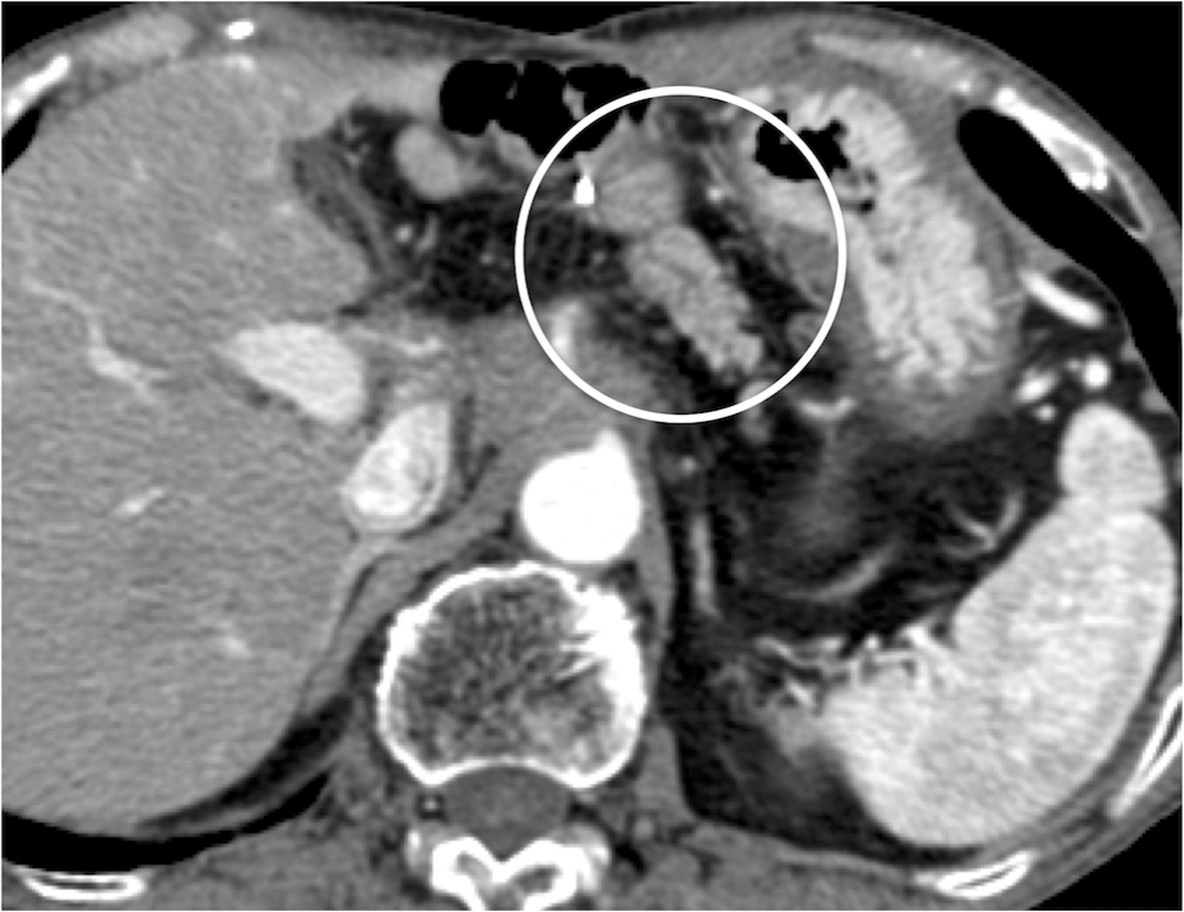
Follow-up CT at 1 year after procedure showed no varices at a portion of the PJ
